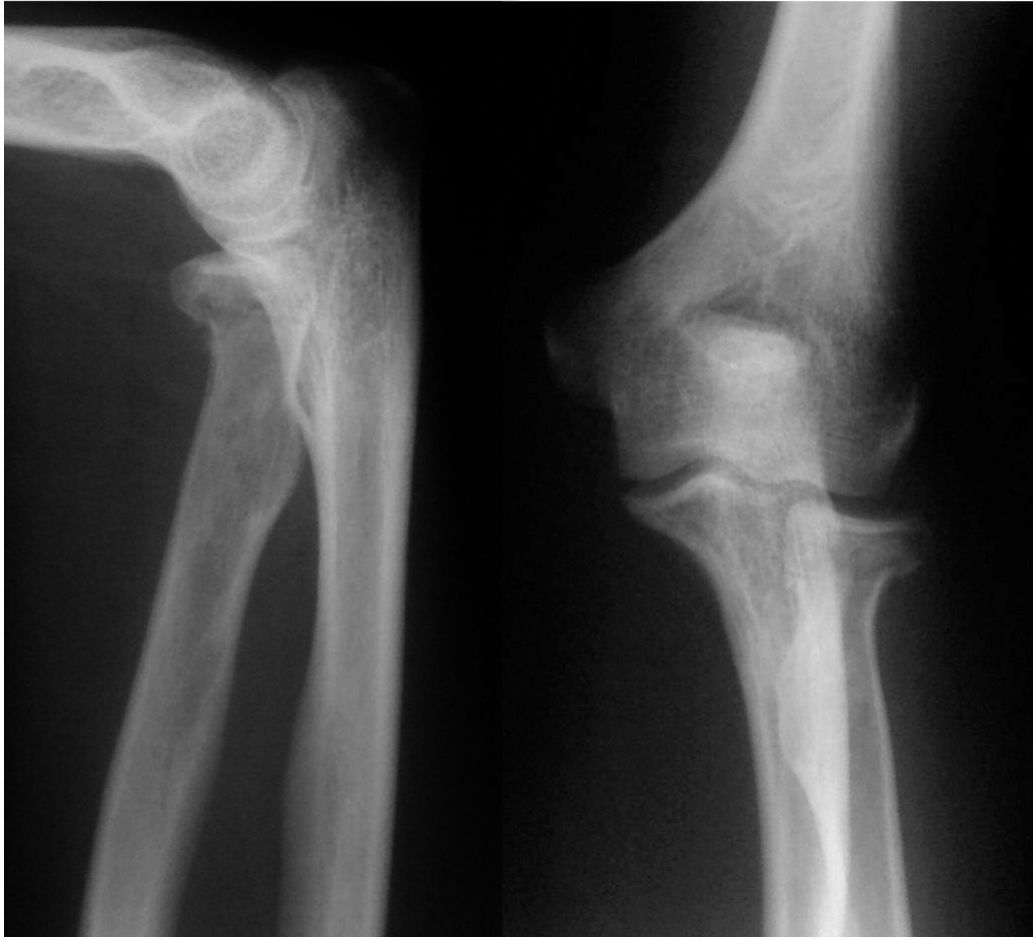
Initial presentation AP and lateral radiographs of a 27-year-old male who sustained a Mason type-I fracture of the left elbow while playing sport. An Essex-Lopresti lesion was diagnosed at 2 weeks when bilateral wrist radiographs were taken. Management was conservative, using collar and cuff immobilization for 1 week followed by supervised physiotherapy. At 6 months, the patient had a flexion arc of 152 degrees and a forearm rotation arc of 180 degrees, with an excellent MES (100).
